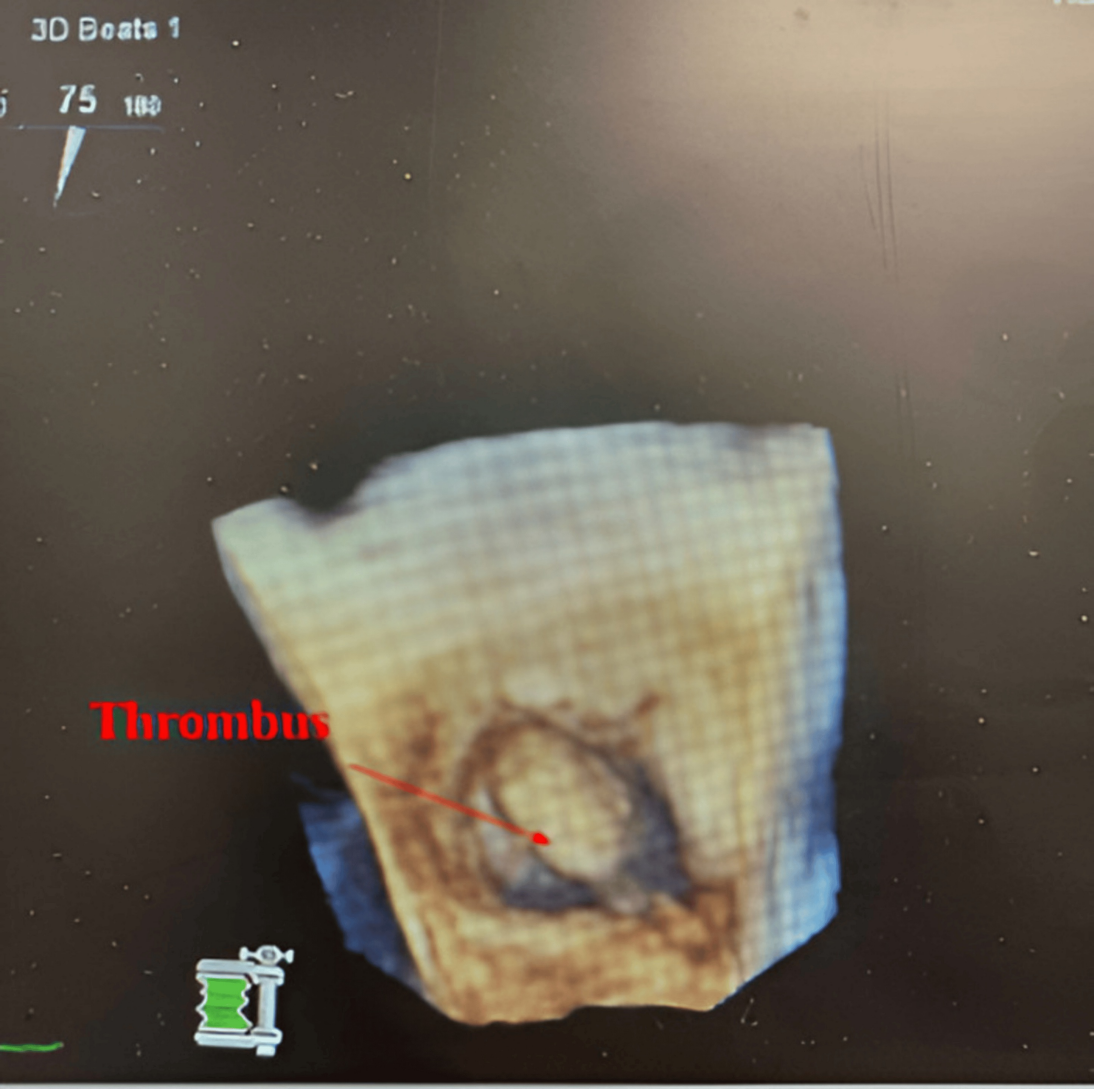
Transesophageal echocardiogram - zoomed view in 3D mode demonstrating the left atrial appendage thrombus measuring 8 x 15 mm with clear visualization of its attachment point and mobility.
